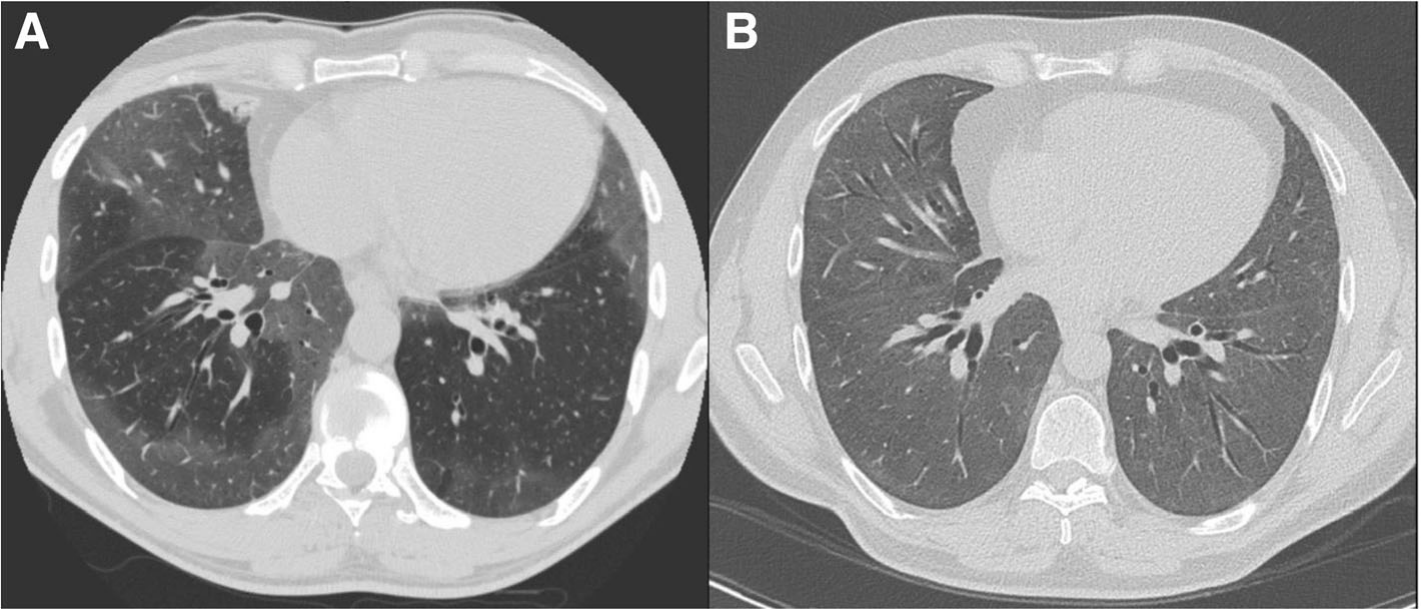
High-resolution computed tomography (HRCT) of the chest in axial cut from time of initial presentation (a), and 2 years post-diagnosis (b). Both HRCTs demonstrate multifocal ground glass consolidation, primarily in the lower lung zones, progressive over an 8-year period
